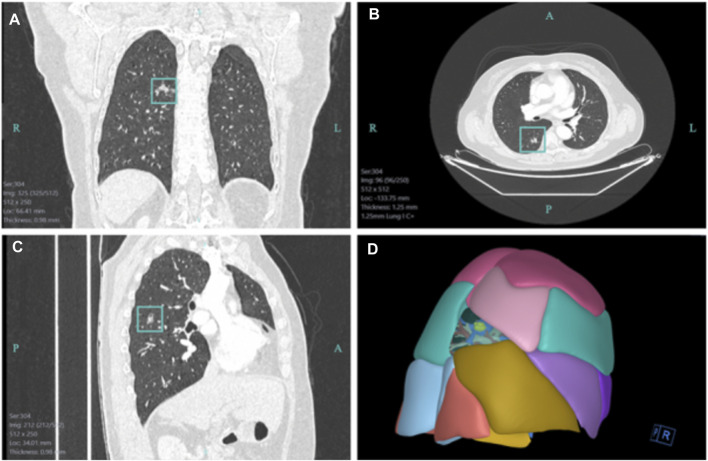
The 3D reconstruction image based on the 2D CT image in the First Hospital of Jilin University. (A) Coronal view of a 2D CT lesion. (B) Transverse view of a 2D CT lesion. (C) Sagittal view of a 2D CT lesion. (D) Three-dimensional reconstruction image based on the 2D CT lesion.
